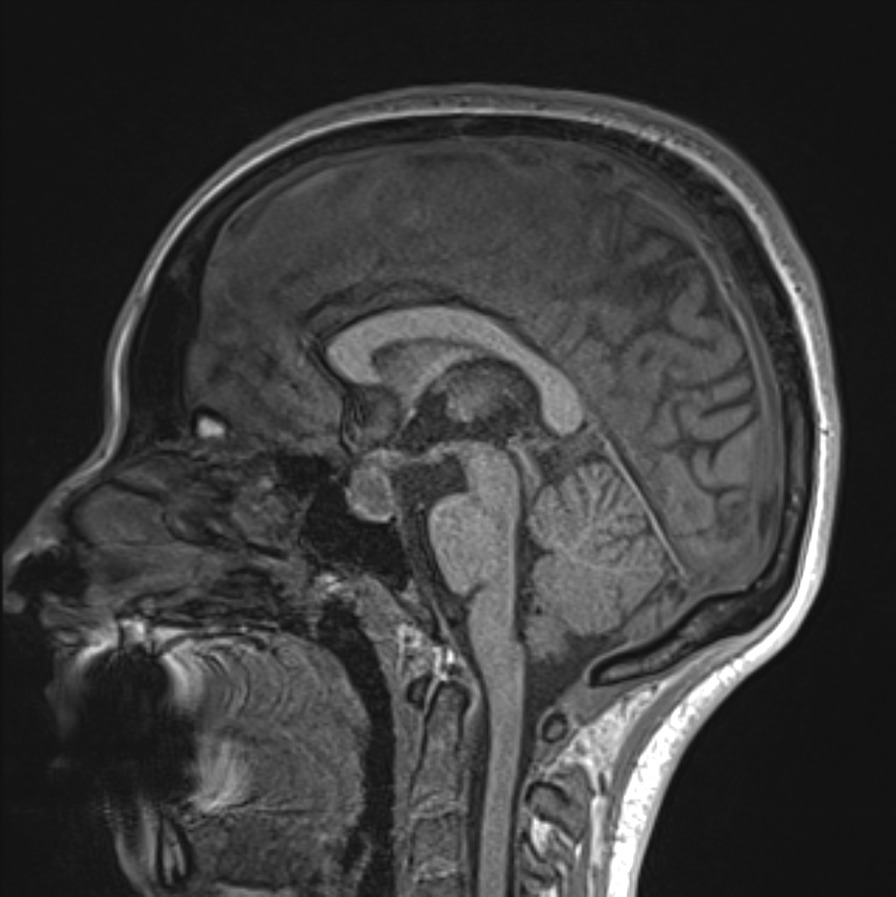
Pre-operative MRI brain scan sagittal T1 sequence showing a hypointense pituitary mass
